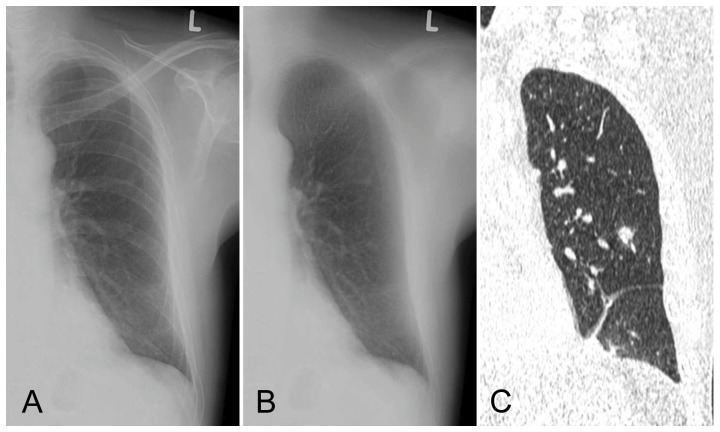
Upright radiograph with a very subtle lesion in the left lower lobe. Without BSI only one observer marked the small lesion. With BSI all 7 observers marked the lesion with an average suspiciousness score of 55. Original radiograph (A), bone suppressed image (B), coronal CT slice (C).
